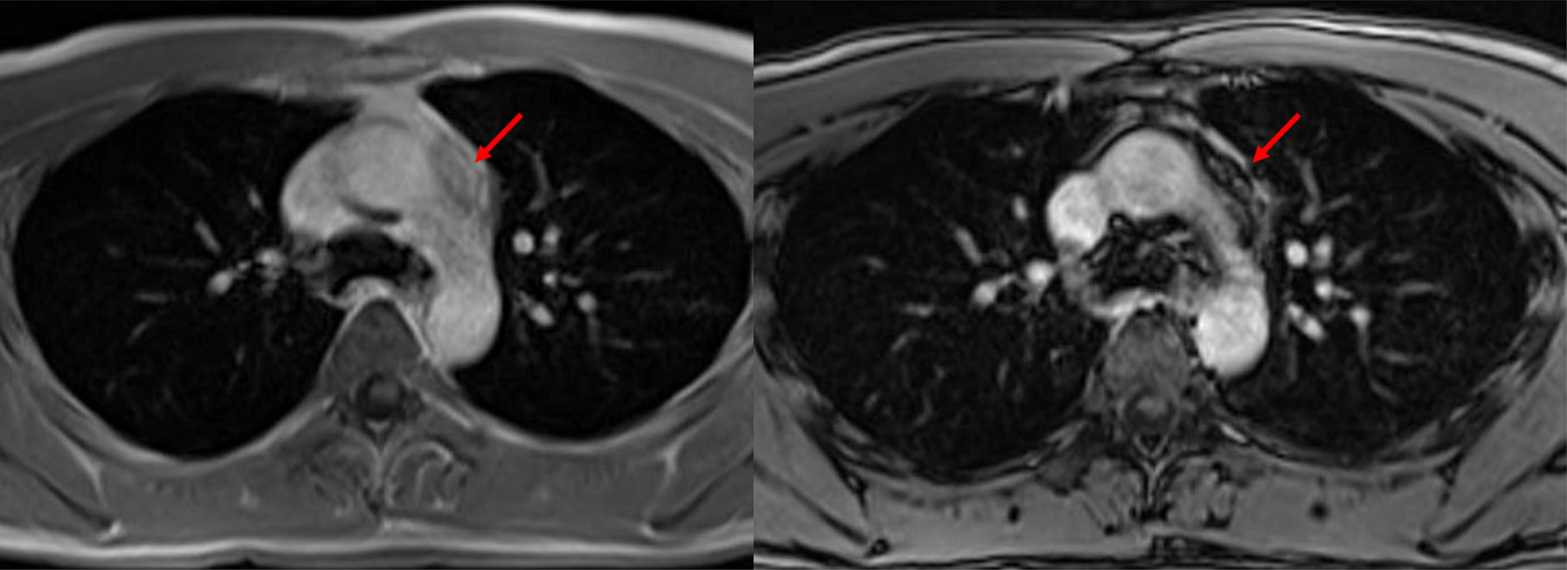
Sclerosing thymoma. A 27-year-old male patient with sclerosing thymoma (arrow). Placing a ROI in the same area on in-phase (left) and opposed-phase (right) T1-weighted images to identify SII, which was 64.6%.
